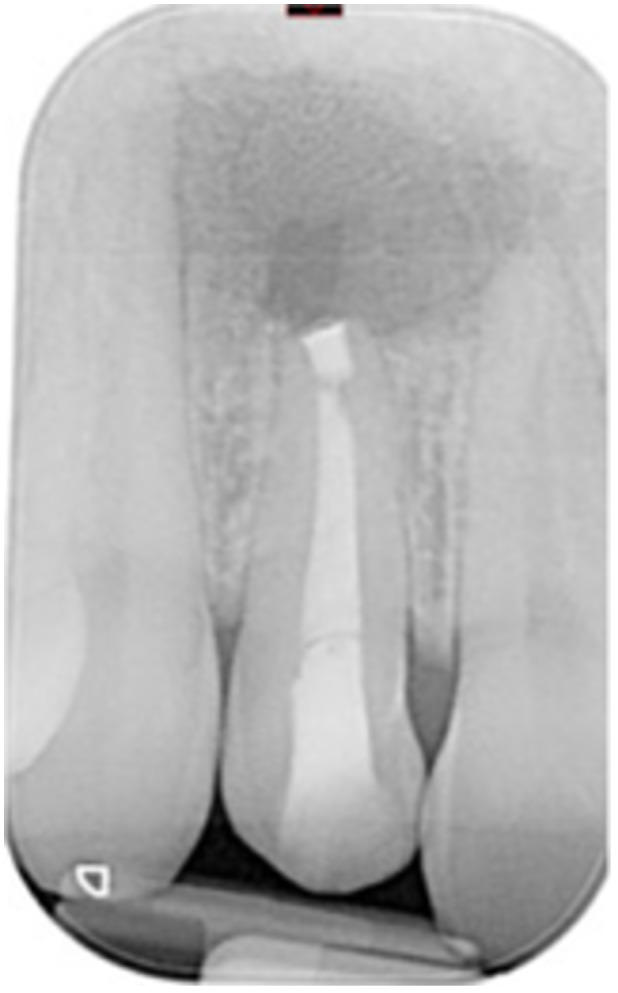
Case 7: The preoperative CBCT and periapical radiograph show an extensive periapical radiolucency associated with tooth 12, which has extended to teeth 11 and 13. Apical surgery was performed on tooth 12 only, as teeth 11 and 13 were deemed vital. At 6 months, marked healing can be seen, evidenced by a reduction in the periapical radiolucency size associated with tooth 12.
